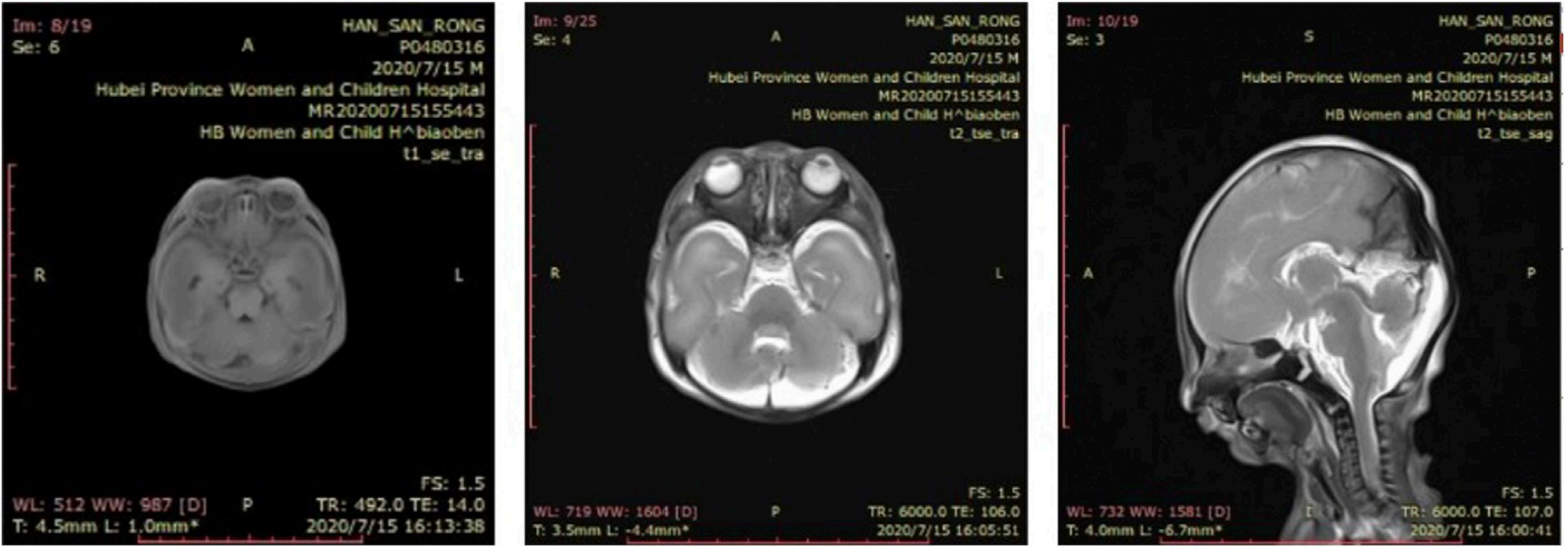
Brain imaging. Cranial MRI showed small lower jaw, displacement of the tough toward the back of the oral cavity and cleft palate and lissencephaly. There was no gyri/sulci clearly seen for both cerebral and cerebellum cortex. (A) T1-weighted coronal image (B) T2-weighted coronal image (C) T2-weighted sagittal image.
